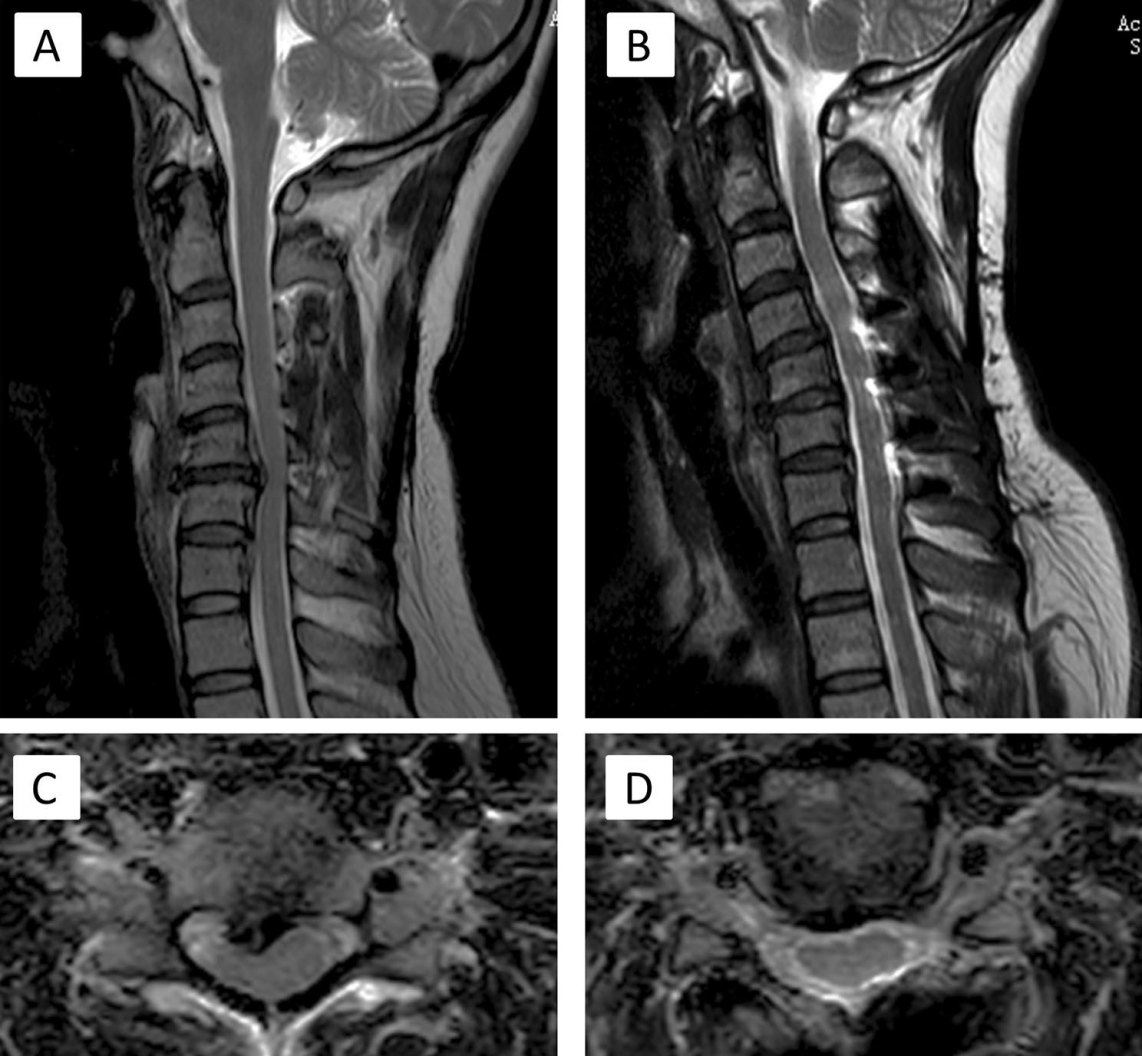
One patient with single-level myelopathy and loss of cervical lordosis showing a complete resolution of CDH. The sagittal preoperative MRI showed the C5/6 disc herniation (A,C) with single-level myelopathy. Then this patient accepted CMEL treatment. After 15 months, the MRI image indicated the profound regression of CDH (B,D).
